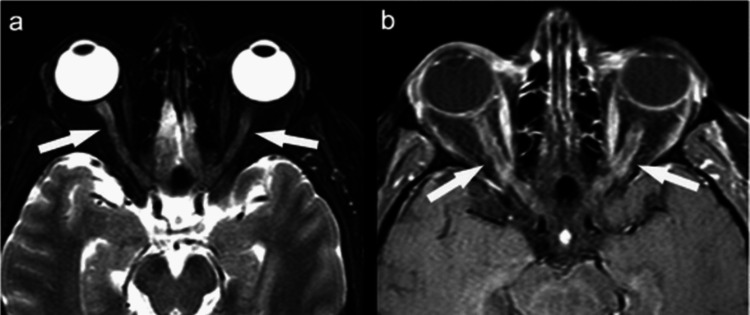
Contrast-enhanced MRI of the orbits showing enhancement of the anterior part of both optic nerves
